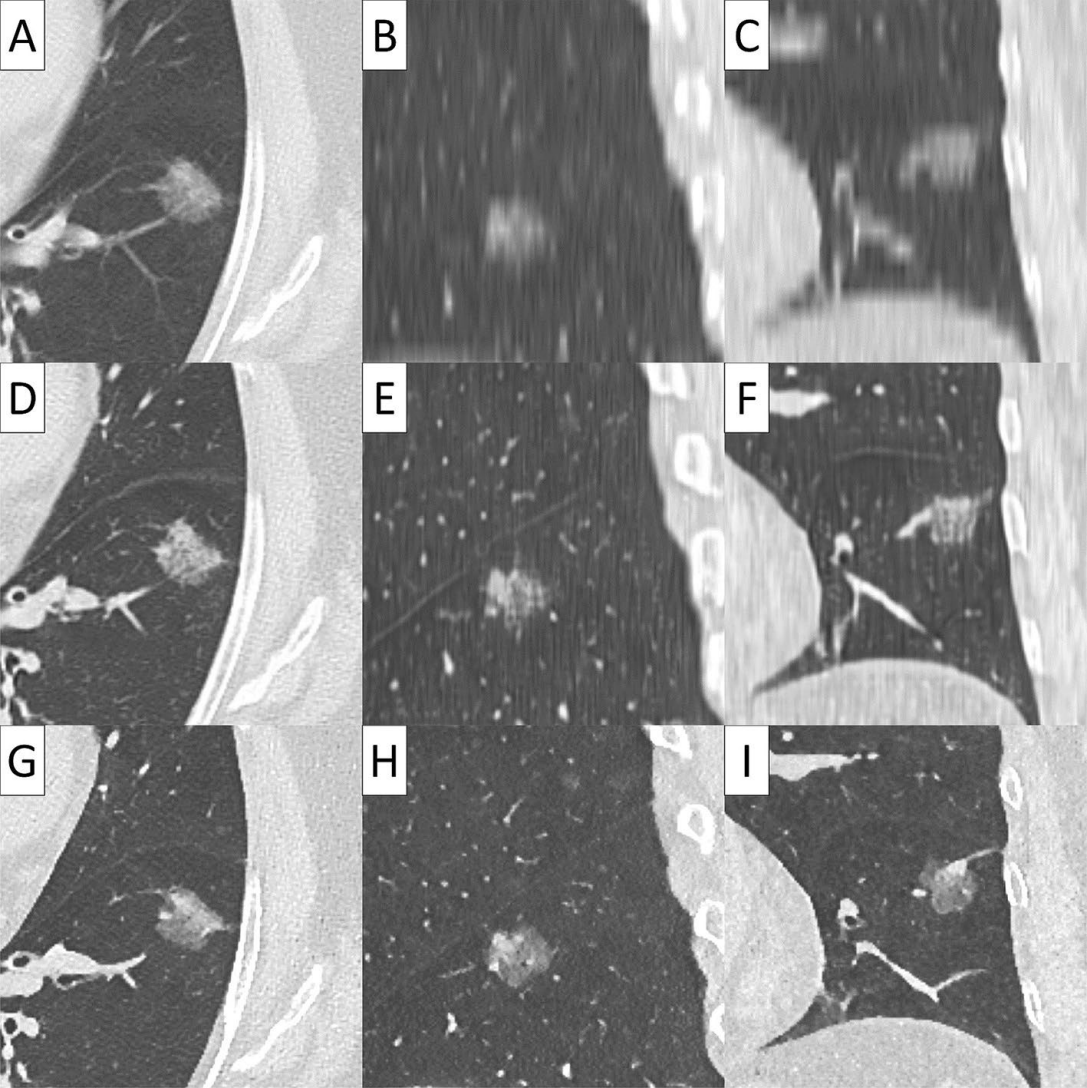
Papillary adenocarcinoma in a 72-year-old female. (A) Axial view, (B) sagittal view, and (C) coronal view of conventional 5-mm thick CT. (D) Axial view, (E) sagittal view, and (F) coronal view of virtual thin-section CT (TSCT). (G) Axial view, (H) sagittal view, and (I) coronal view of real TSCT.
